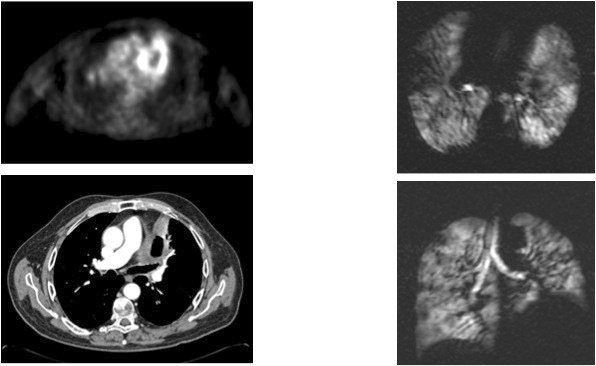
Response after XRT – cavitation is seen on CT and response on PET. Interestingly obstruction nearly completely resolved on Helium MRI.
